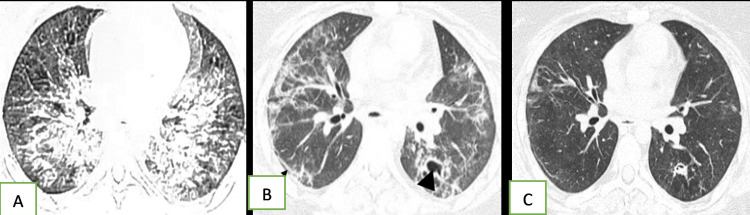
A 23-year-old female with severe COVID-19 Initial CT shows extensive bilateral consolidation and ground-glass opacities (GGOs), predominantly in central distribution (A). The first follow-up CT after 34 days of diagnosis shows mixed reticular and GGOs, as well as parenchymal bands (thin black arrow) mainly in peripheral distribution; an irregular cavity has formed in the left lower lobe (short black arrow) (B). Second follow-up CT after 85 days of diagnosis shows significant resolution of all opacities, as well as reduction in the cavity size (C).
